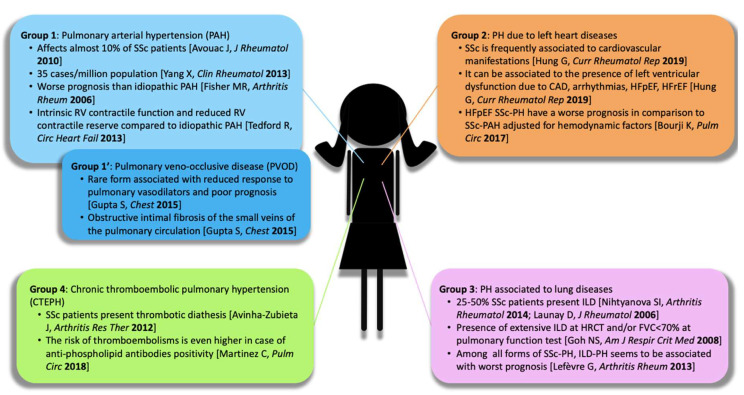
Different phenotypes of pulmonary hypertension in patients with systemic sclerosis. Abbreviations: PAH: pulmonary arterial hypertension; SSc, systemic sclerosis; RV, right ventricle; PVOD, pulmonary veno-occlusive disease; PH, pulmonary hypertension; CAD, coronary artery disease; HFpEF, Heart failure with preserved ejection fraction; HFrEF, heart failure with reduced ejection fraction; CTEPH, chronic thromboembolic pulmonary hypertension; ILD, interstitial lung disease; HRCT, high resolution computed tomography; FVC, forced vital capacity.
